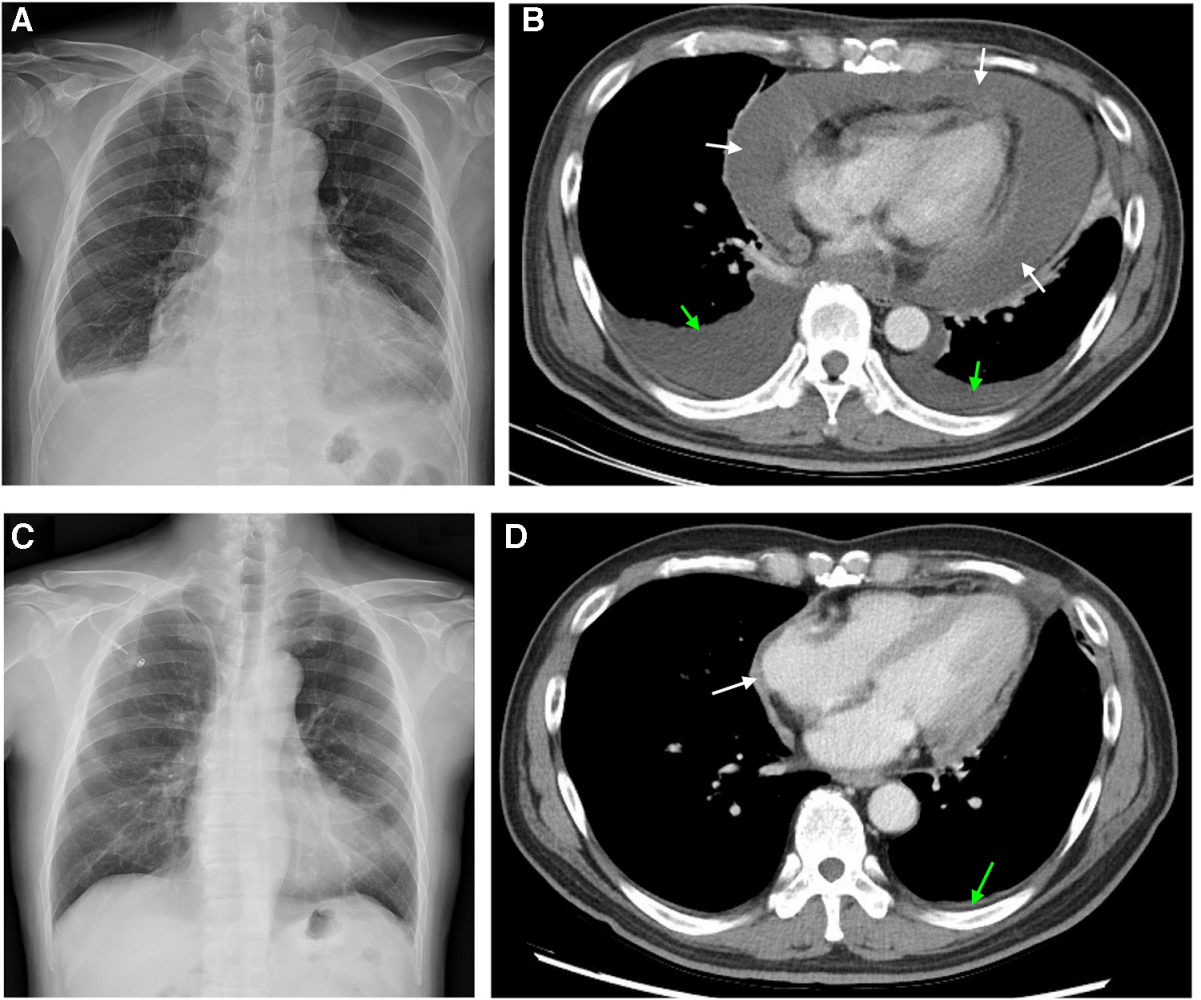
Chest image findings before and after 6 cycles of chemotherapy. (A) x-ray image shows cardiomegaly with pericardial effusion and blunted left costophrenic angle. (B) Computed tomography image shows pericardial effusion with noticeable pericardial thickening and bilateral pleural effusion. (C) x-ray image showed normal heart size without pleural effusion. (D) Computed tomography image showed subsiding pericardial effusion and lessened pericardial thickening. White and green arrows indicate the areas of pericardial and pleural effusion, respectively.
